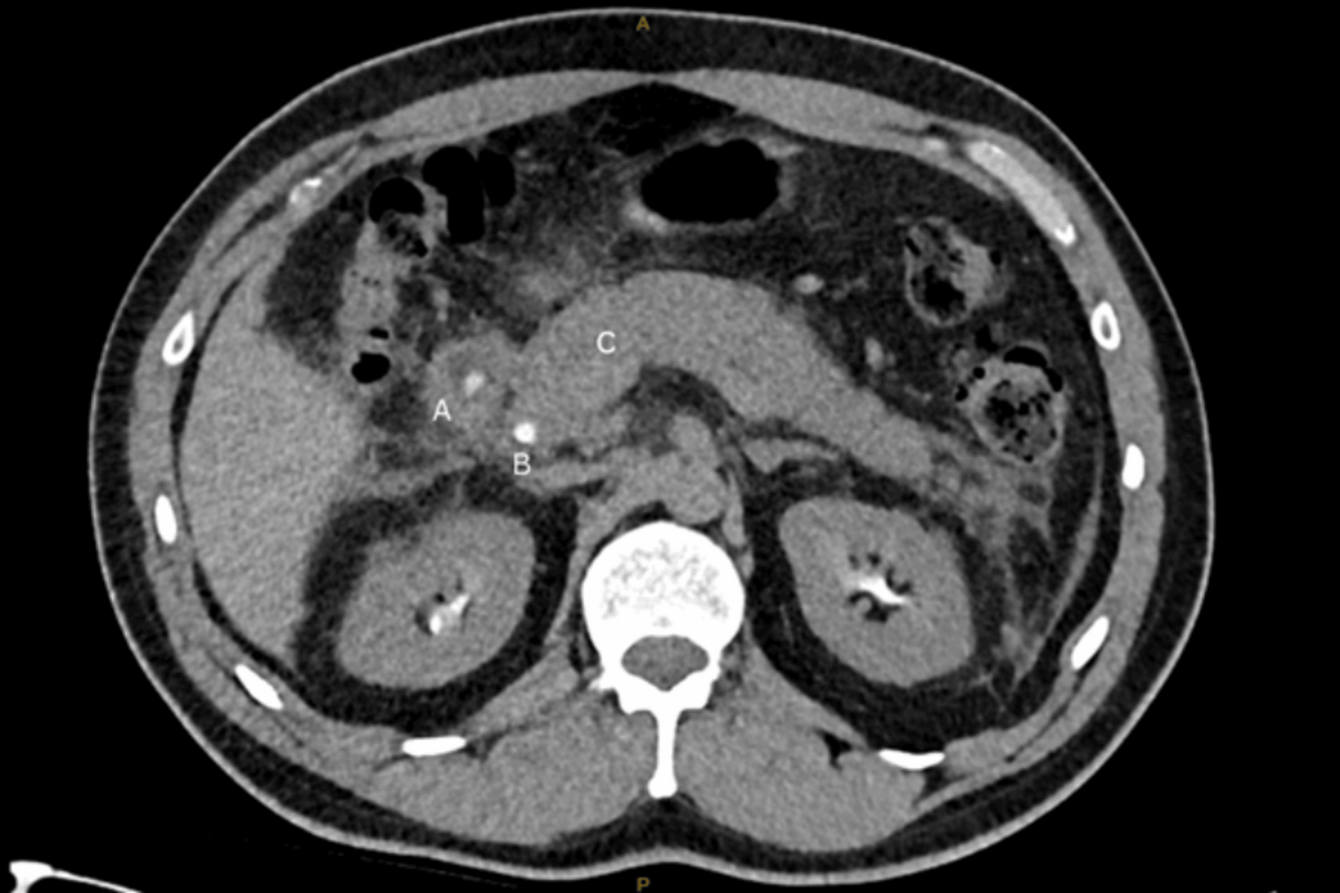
CT cholangiogram (axial view) on postoperative day 1 A: contrast in the duodenum, B: contrast in the common bile duct, C: mild fat stranding around the pancreas, suggestive of acute pancreatitis
